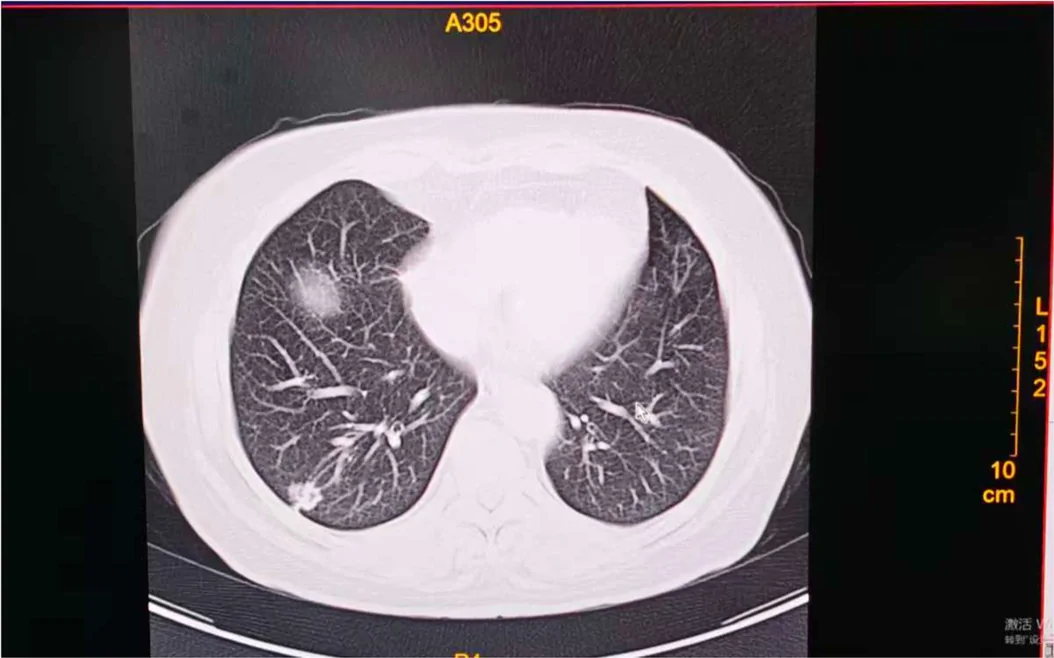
Results of Chest CT The image on the left shows the preoperative visible mass. The picture on the right is 6 months after surgery.
